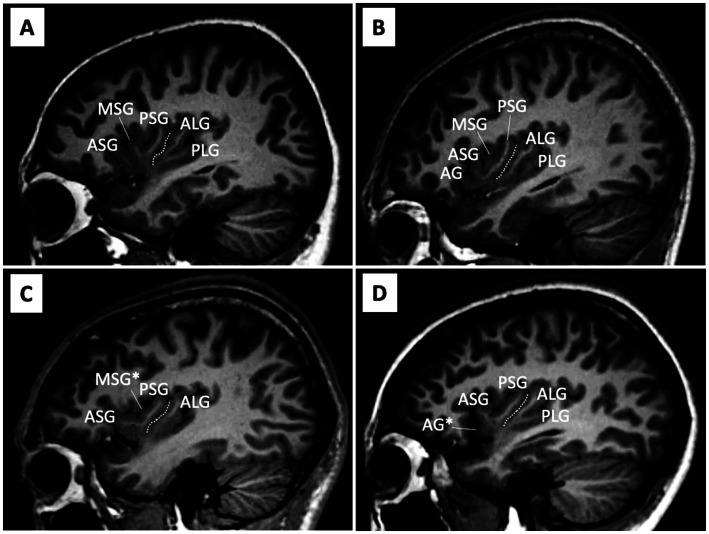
Anatomical variations in the insular cortex on sample MR images in the sagittal view. (A-D) Assessments of gyral development were mainly performed using consecutive sagittal slices, with references to simultaneously displayed coronal and axial views. The central insular sulcus (white dotted lines) subdivides the anterior (short insula) and posterior (long insula) subdivisions. The AG and MSG had large inter-individual variations in the degree of development [i.e., absent, underdeveloped (marked with an asterisk), or well-developed], while the ASG, PSG, and ALG were well-developed in all hemispheres. The PLG was generally present, but was absent in some hemispheres (e.g., hemisphere C). AG, accessory gyrus; ALG, anterior long gyrus; ASG, anterior short gyrus; MSG, middle short gyrus, PLS, posterior long gyrus; PSG, posterior short gyrus.
